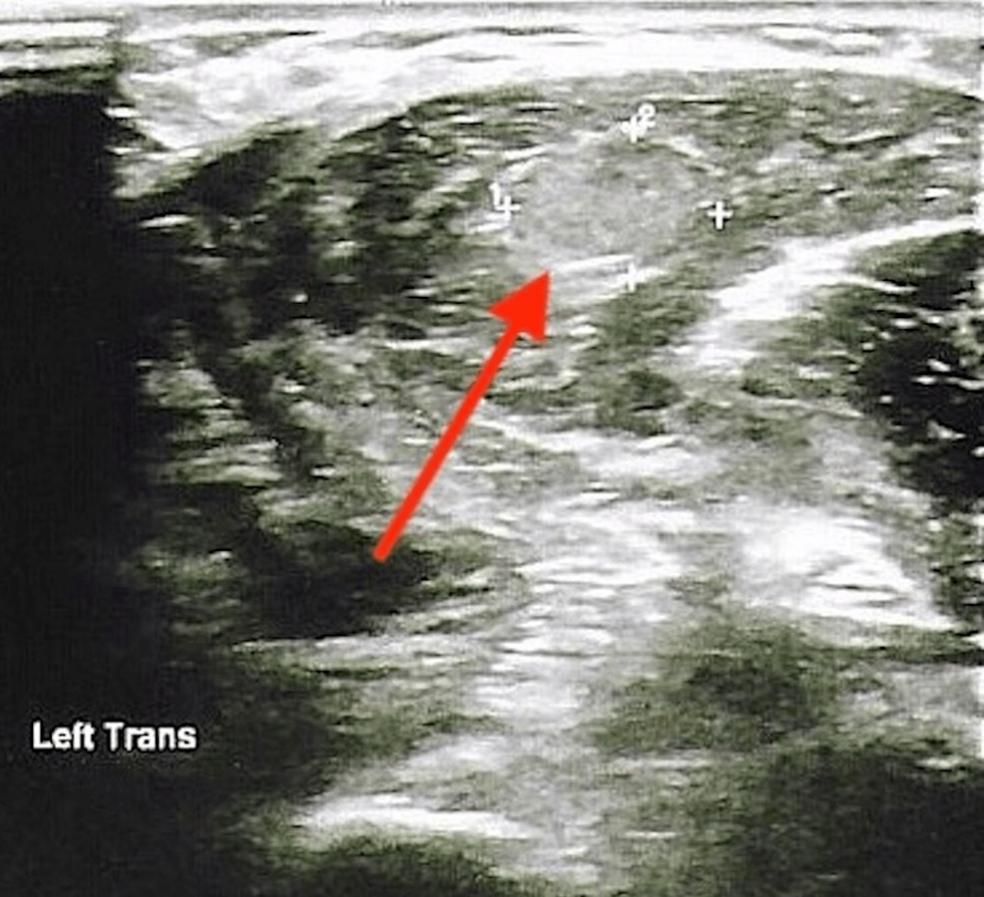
Left forearm ultrasound transverse view revealing an auto-transplanted parathyroid adenoma (red arrow)
